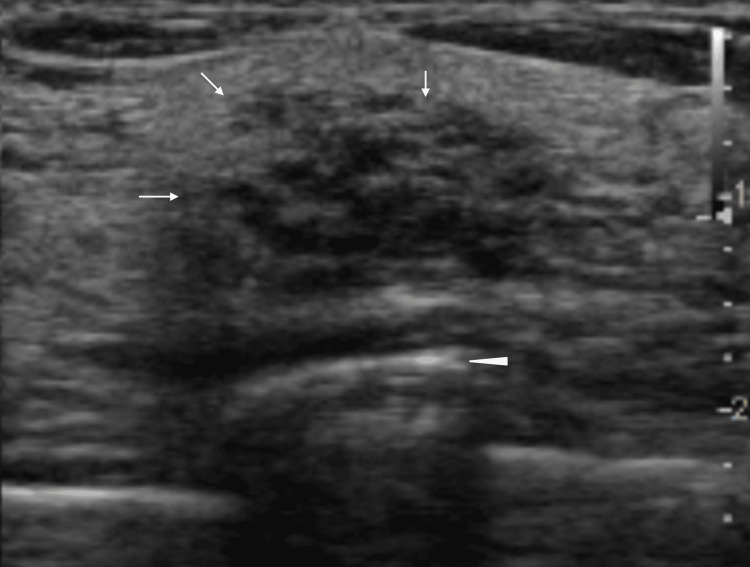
Ultrasound findings Ultrasound showed that the mass had indistinct margins (arrows), hyperechoic dots/lines, and slight posterior acoustic enhancement (arrowhead).
